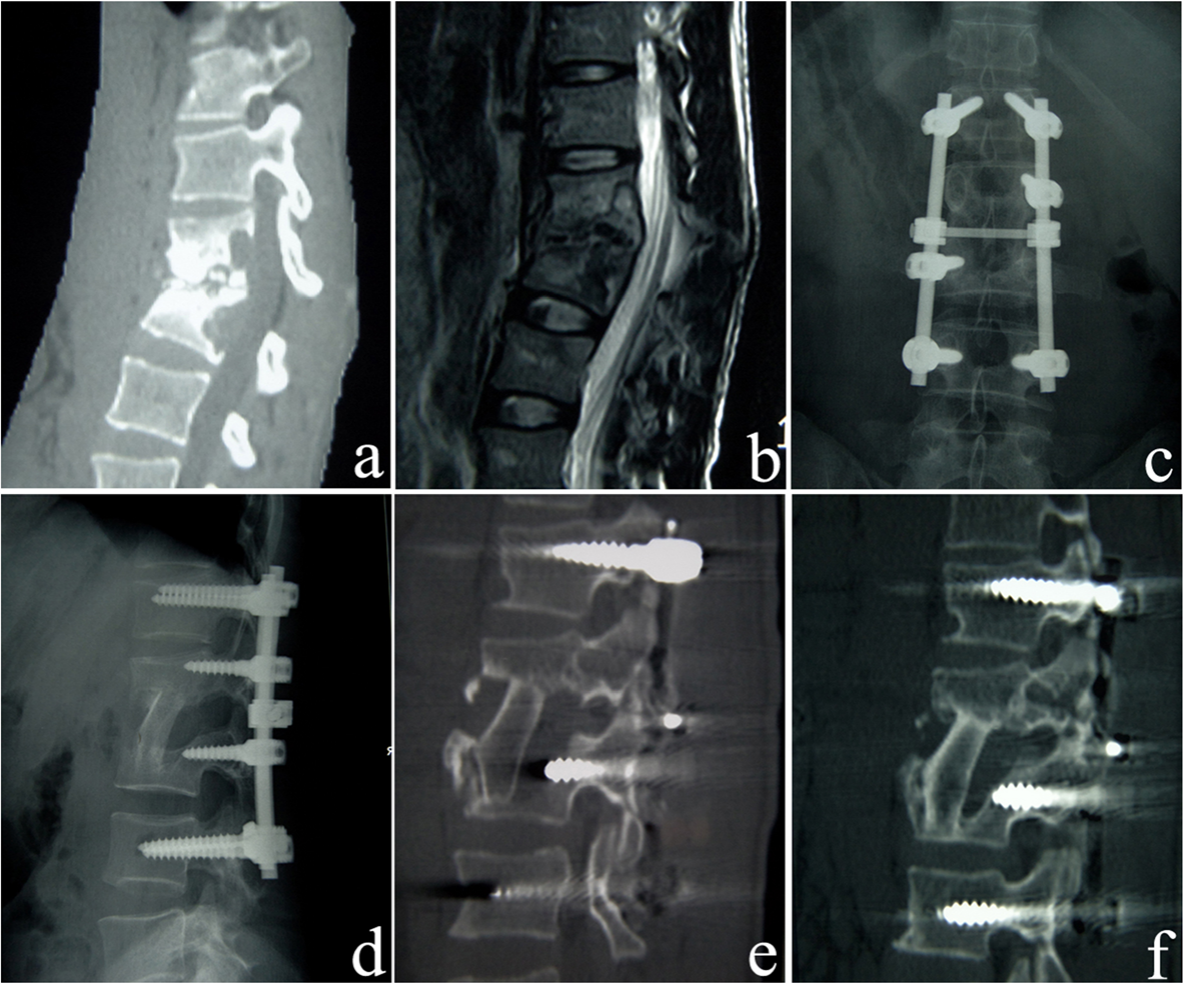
A 25-year-old male who underwent anterior-posterior surgery and short-segment fixation. a Preoperative sagittal CT reconstruction shows destruction of L2 and L3 vertebrae and narrowing of the L2-L3 intervertebral space. b. The preoperative sagittal contrast-enhanced MRI shows confounding signals in L2 and L3 vertebrae, destruction of the L2-L3 intervertebral space, and nerve compression. c, d Postoperative X-ray anteroposterior image shows that the strut bone is located firmly between the affected vertebrae, the short-segment fixation is excellent, and the L2 and L3 vertebrae are fixed with short pedicle screws. e Three months after surgery, the sagittal CT reconstruction shows that the lesion of L2 and L3 vertebrae is removed completely, and the strut bone is located firmly. f Five years after surgery, the sagittal CT reconstruction shows healing of L2 and L3 tuberculosis lesions and bone graft fusion
